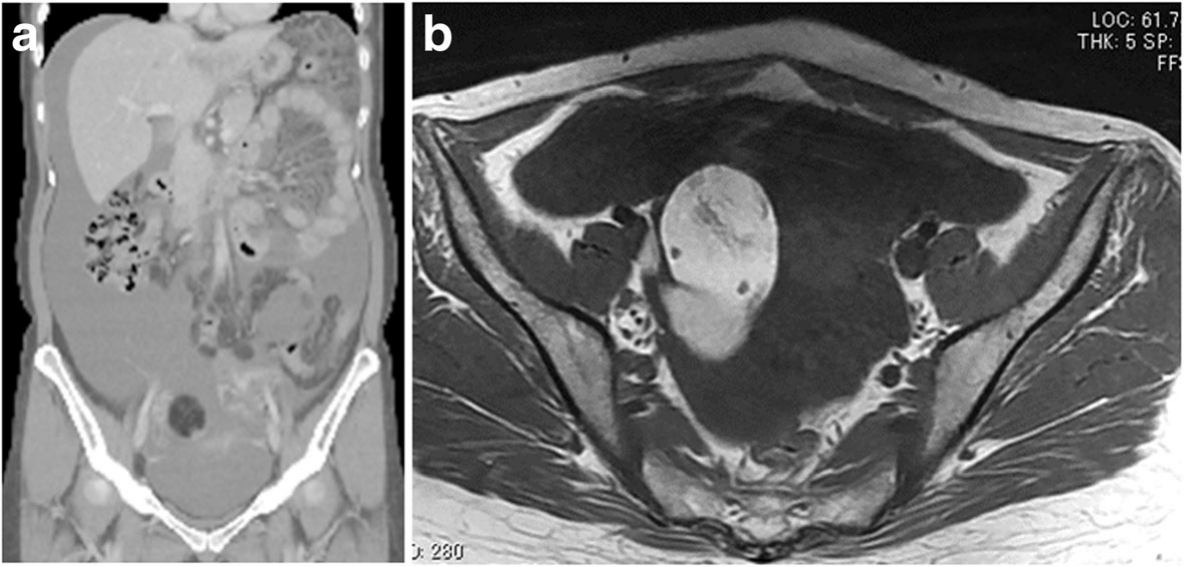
a Contrasted computed tomography showed distended abdominal cavity filled with low-density fluid. b A pelvic magnetic resonance image of the right ovarian mass with high signal intensity on T2-weighted image
